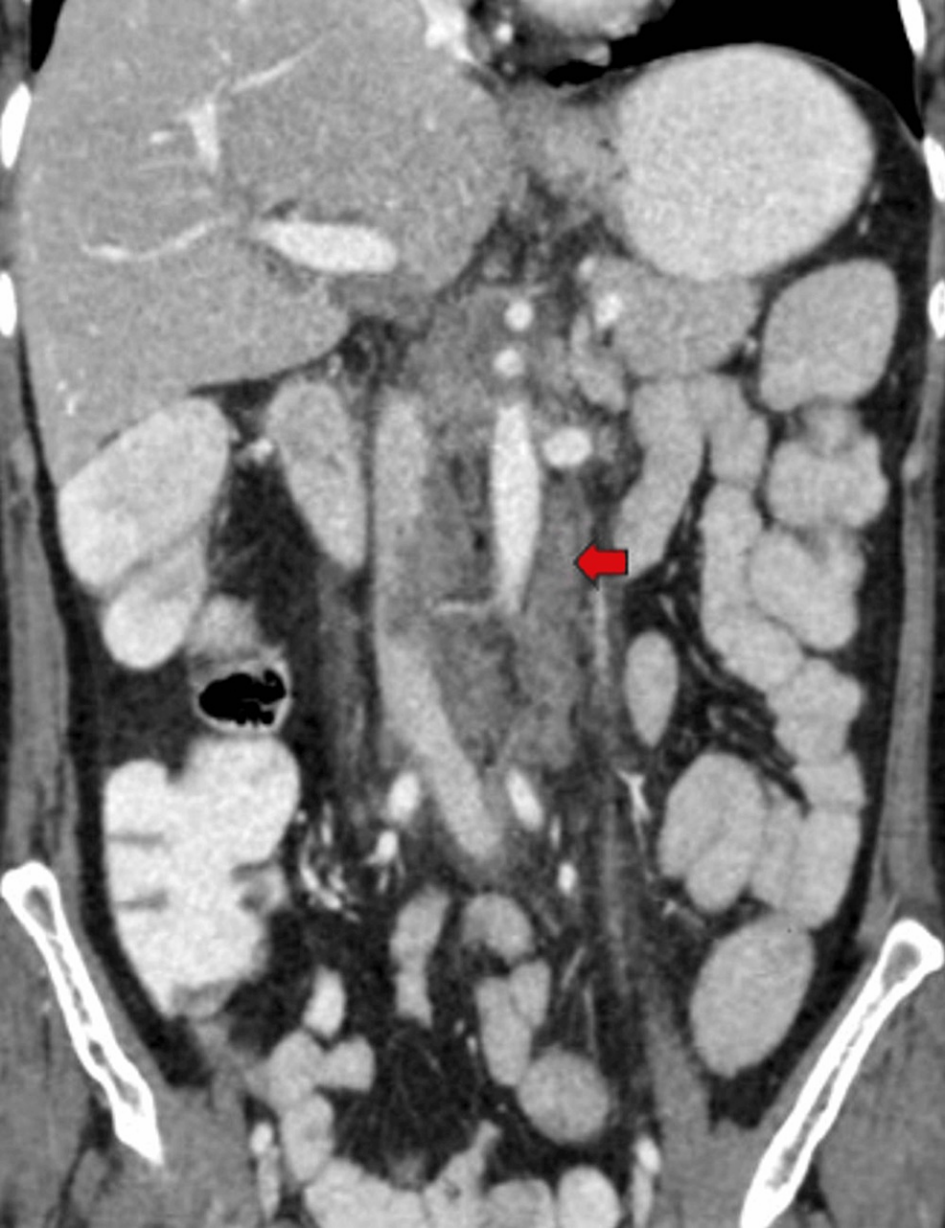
Post-contrast coronal reformatted CT image show enlarged and conglomerated lymph nodes seen in the para-aortic (red arrow) and aorto-caval regions with central hypodense nonenhancing necrotic areas. CT: Computed tomography
